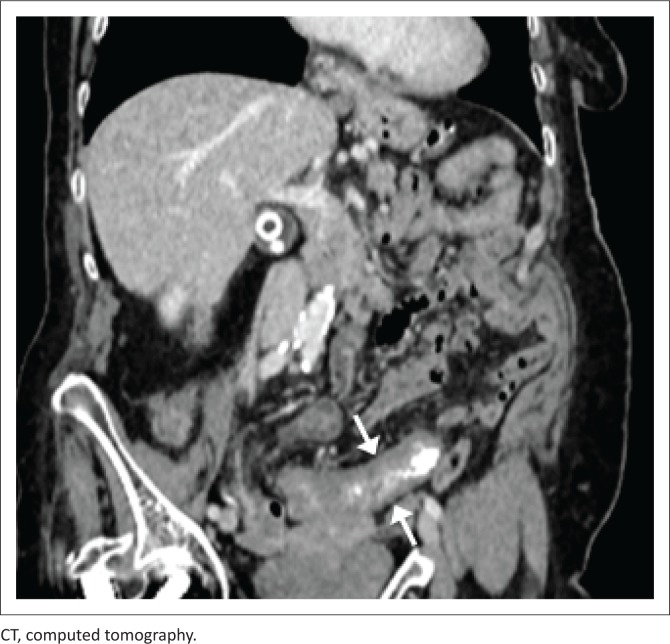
Coronal reconstruction of portovenous phase–enhanced CT, of the above patient, again demonstrating circumferential mural thickening of the sigmoid colon.
